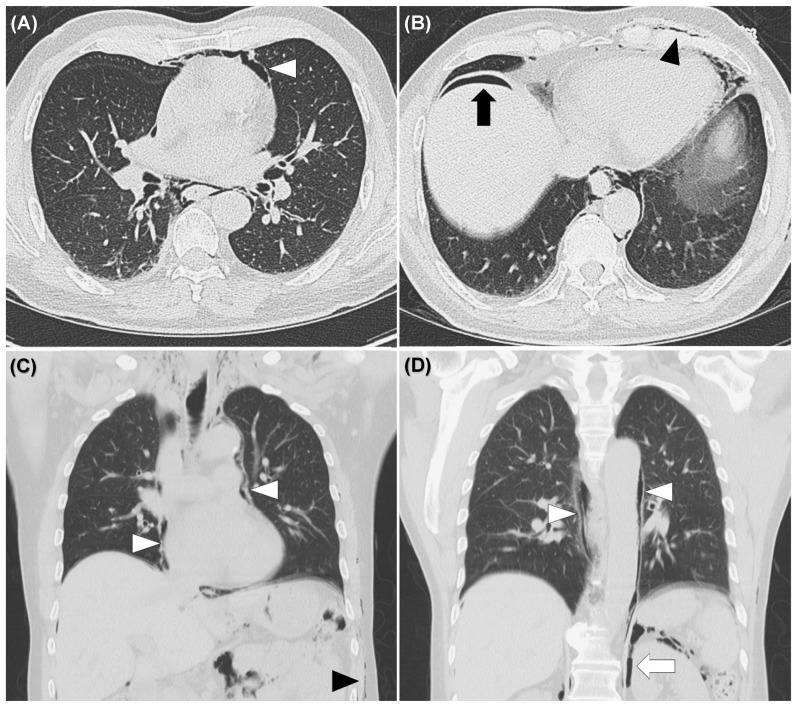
Non-enhanced computed tomography of the chest showed pneumomediastinum (white arrowheads) (A,C,D), subcutaneous emphysema (black arrowheads) (B,C), pneumoperitoneum (black arrow) (B), pneumoretroperitoneum (white arrows) (D), but no evidence of rib fracture and pneumothorax.
